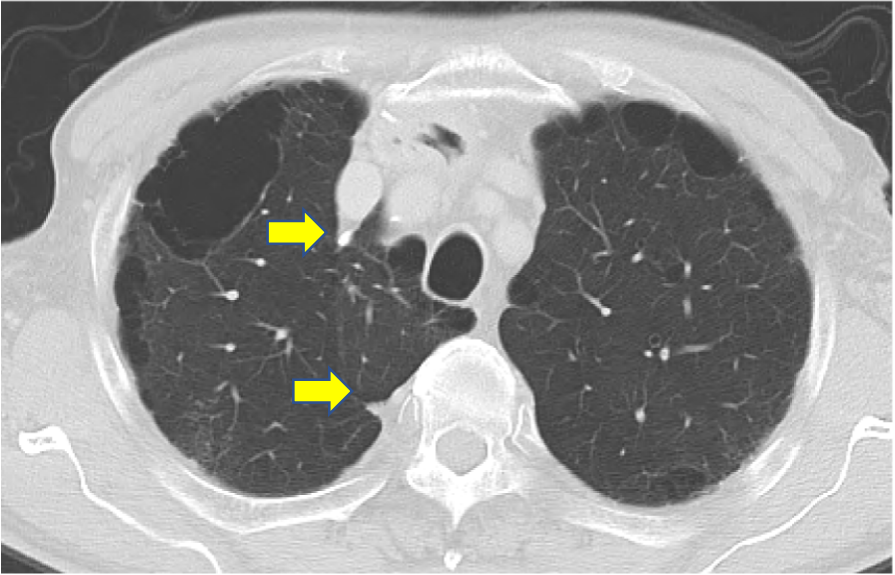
CT showing both ends of the divided azygos vein (arrow). There is no postoperative failure due to the division of the azygos vein for 24 months postoperatively
